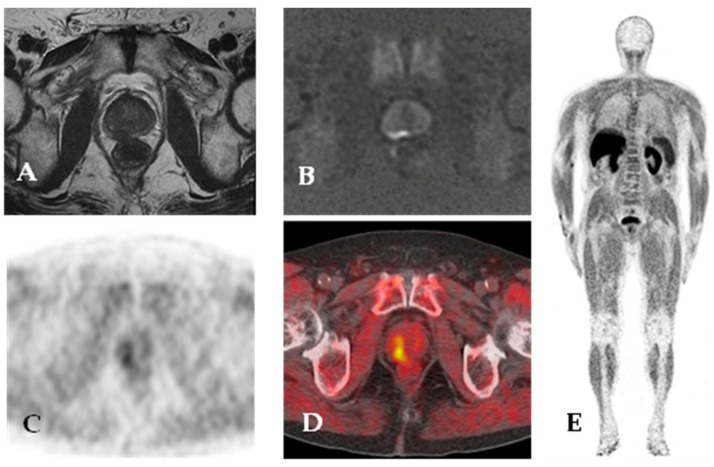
^18^F-choline PET/MR. Staging MR imaging shows a large peripheral zone (right posterior mid-gland) lesion with low signal on T2-weighted images (A) and focal and marked hyperintensity on high b value DWI (B). ^18^F-choline PET/CT images (C,D) show increased tracer uptake in the right posterior area of the prostate gland. Maximum intensity projection (E) ^18^F-choline PET images.
